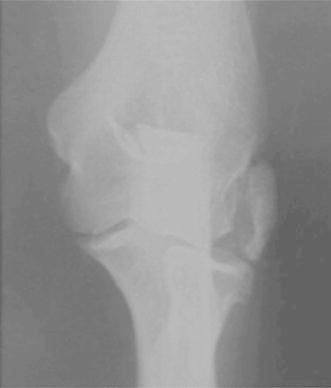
Radiographic examination 8 months after the original injury showing a nonunion of the epicondylar fragment extending distally and communicating with the joint
